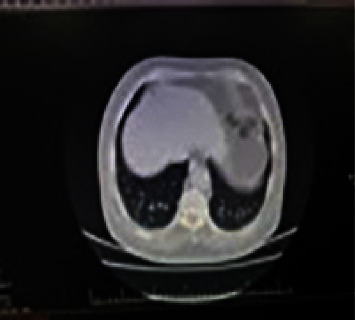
Small subpleural nodule in the right lower lobe (RLL), thoracic CT scan with intravenous contrast.
